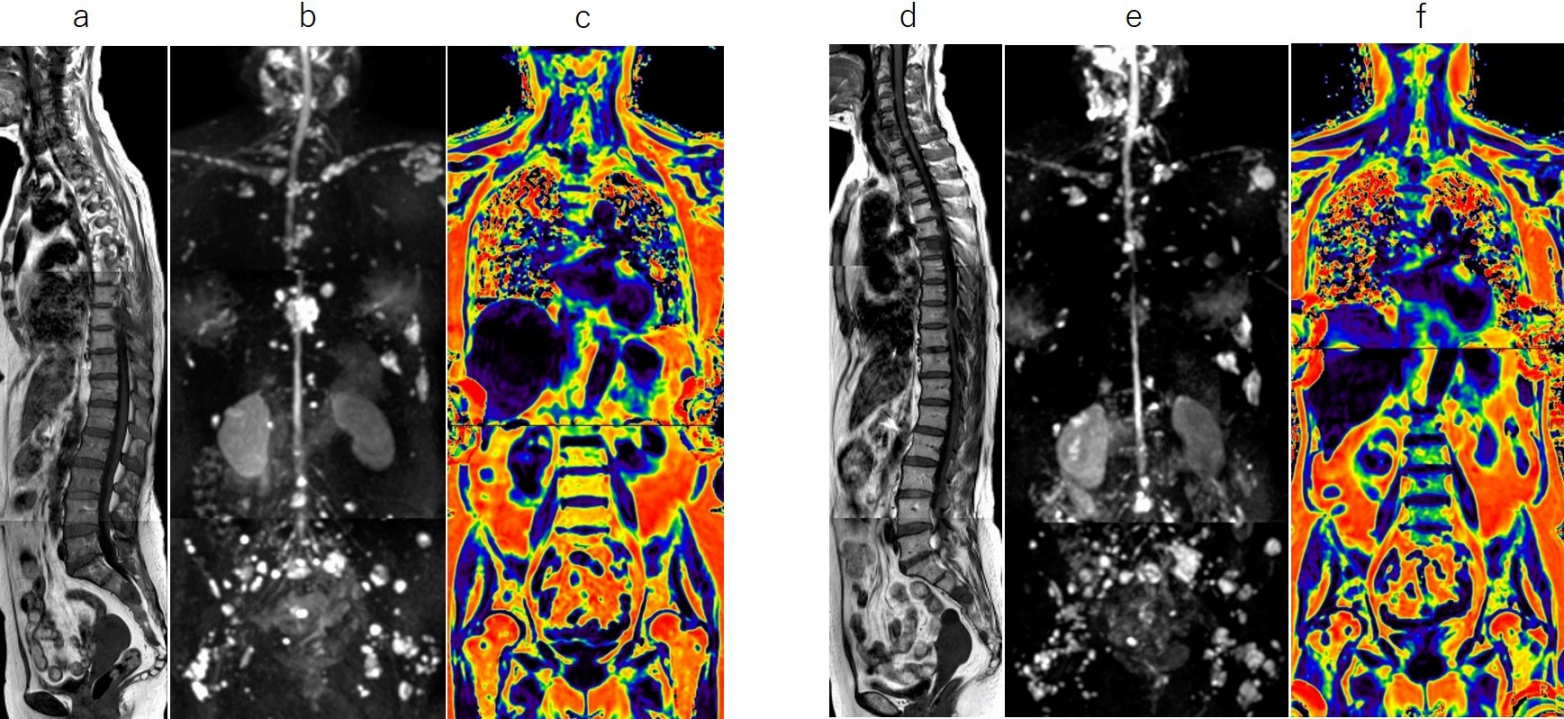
Whole-body MRI with a focal-dominant pattern. Sagittal T1-weighted images (T1WI, a), whole-body diffusion-weighted MRI (WB-DWI, b), and fat fraction (FF) map (c) at baseline, and corresponding T1WI (d), WB-DWI (e), and FF map (f) at 2 cycles of chemotherapy for a 70-year-old woman with symptomatic myeloma. MRI shows numerous focal bone lesions throughout the body at baseline. After 2 cycles of chemotherapy with bortezomib and dexamethasone, total diffusion volume does not show any significant change (from 47 ml to 49 ml), but FF in the lumbar bone marrow has decreased by 30% (from 56% to 40%), indicating an increase in diffuse infiltration into bone marrow by myeloma.
